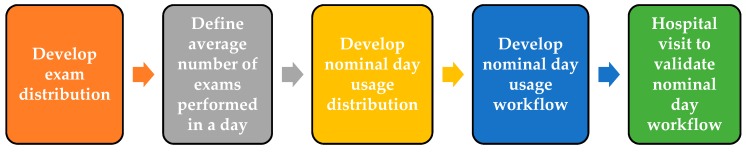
Strategy to develop ‘nominal day usage’ of a magnetic resonance imaging (MRI) system.
